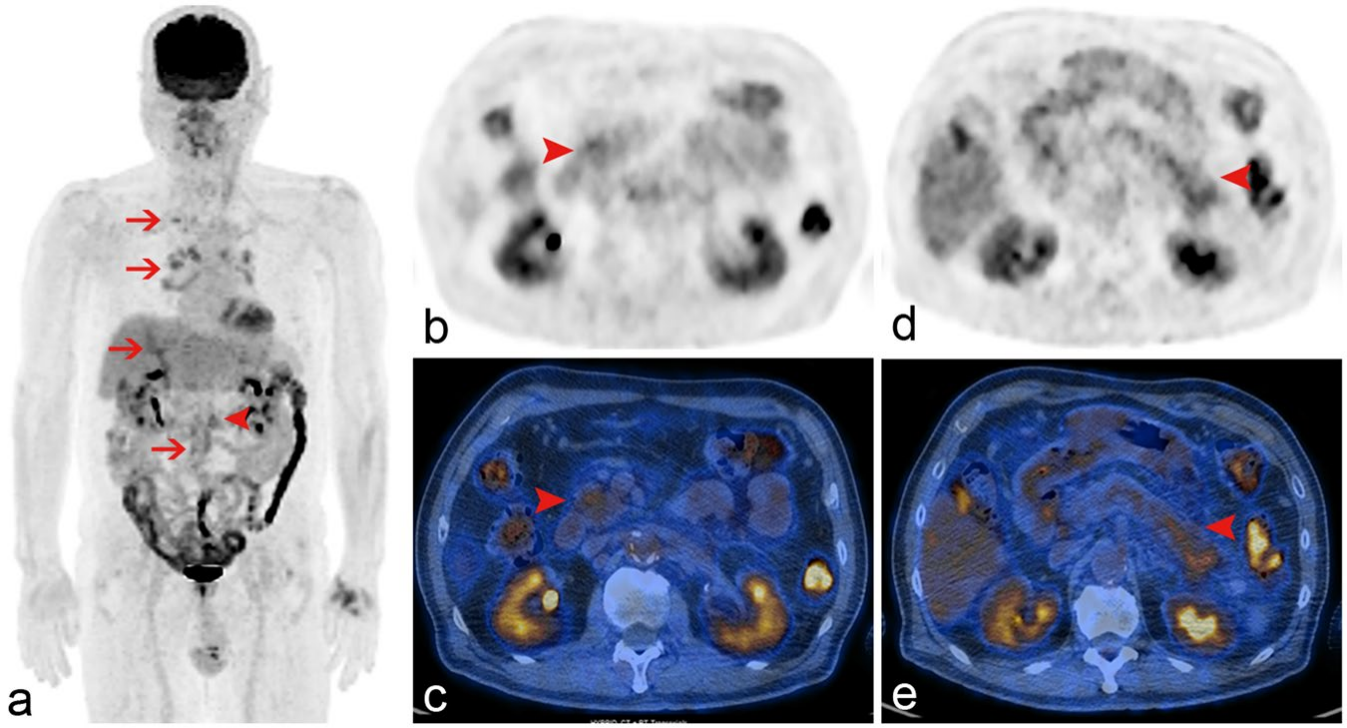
Autoimmune pancreatitis, diffuse type. The maximum intensity projection image (a) revealed more than two foci of extrapancreatic uptake, including left supraclavicular and mediastinal nodes, bile ducts, and abdominal aorta (arrows). Axial (b,d) and PET/CT fused (c,d) images showed patchy diffuse mild to moderate hypermetabolism at pancreatic head to tail (arrowhead). The primary pancreatic tumor exhibited a SUVmax of 6.3 and a low high gray-level zone emphasis value of 94.3.
